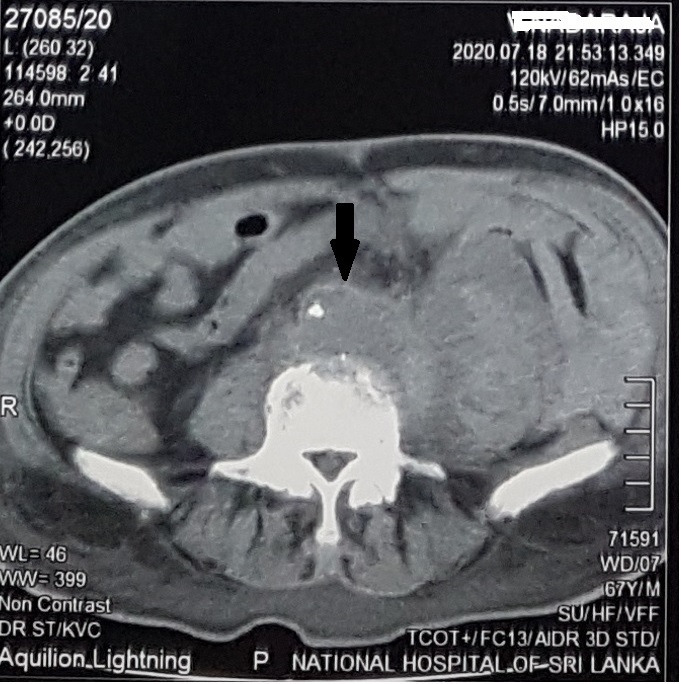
Non contrast CT findings (Arrow indicates the aneurysmal aortic bifurcation).
